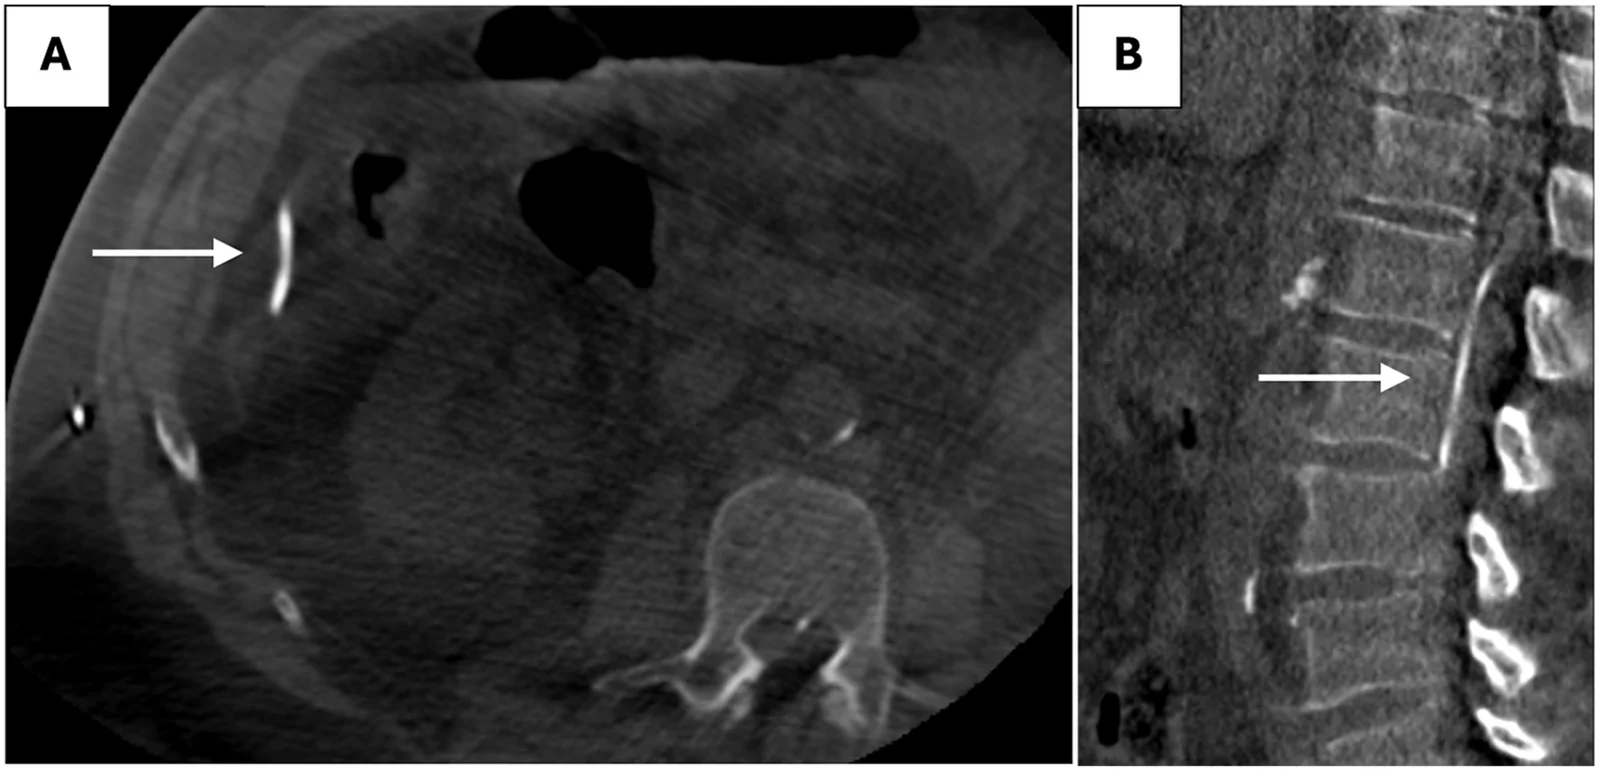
Intraoperative CBCT following LP shunt implantation, demonstrating correct intraspinal course of the lumbar catheter and intraperitoneal positioning of the abdominal catheter (white arrows) captured on a single scan: axial view (A) and sagittal reconstruction (B) of the same dataset.
